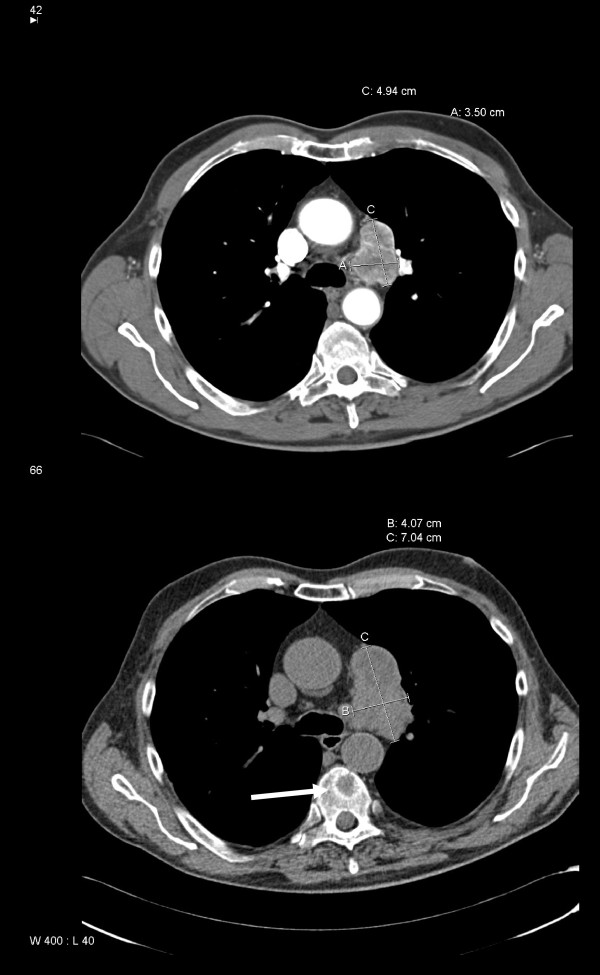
Computed tomography of the chest showing mediastinal lymph node enlargement (upper image: September 2005, i.e. initial diagnosis of metastases). Slow progression in the absence of treatment (lower image: June 2008, i.e. before initiation of sunitinib therapy). The white arrow indicates metastasis in a thoracic vertebra.
